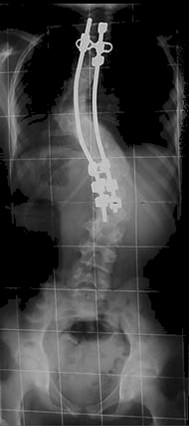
Postoperative standing posteroanterior radiograph showing the levels of instrumentation and the correction of the curve.
